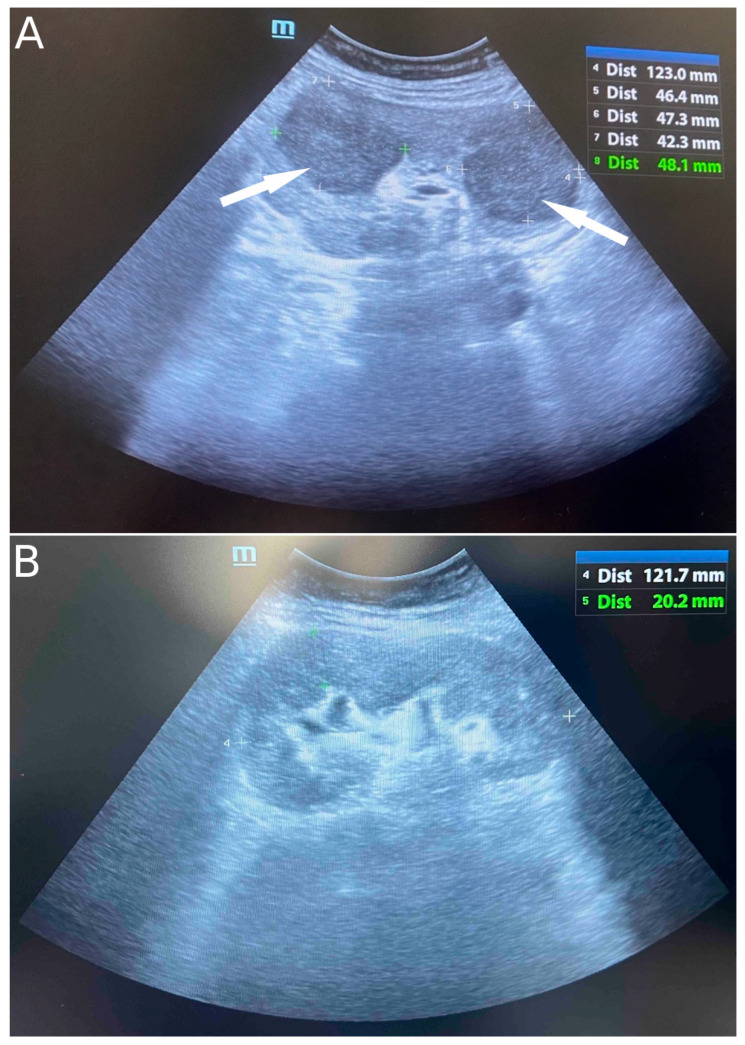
Ultrasound image of infiltrative circular lesions of transplant kidney lymphoma (indicated by arrows) (A) and normal ultrasound image of transplant kidney after six cycles of chemoimmunotherapy (B).
